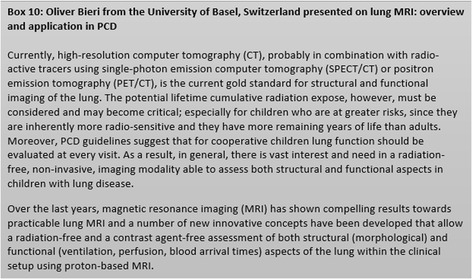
Oliver Bieri from the University of Basel, Switzerland presented on lung MRI: overview and application in PCD
